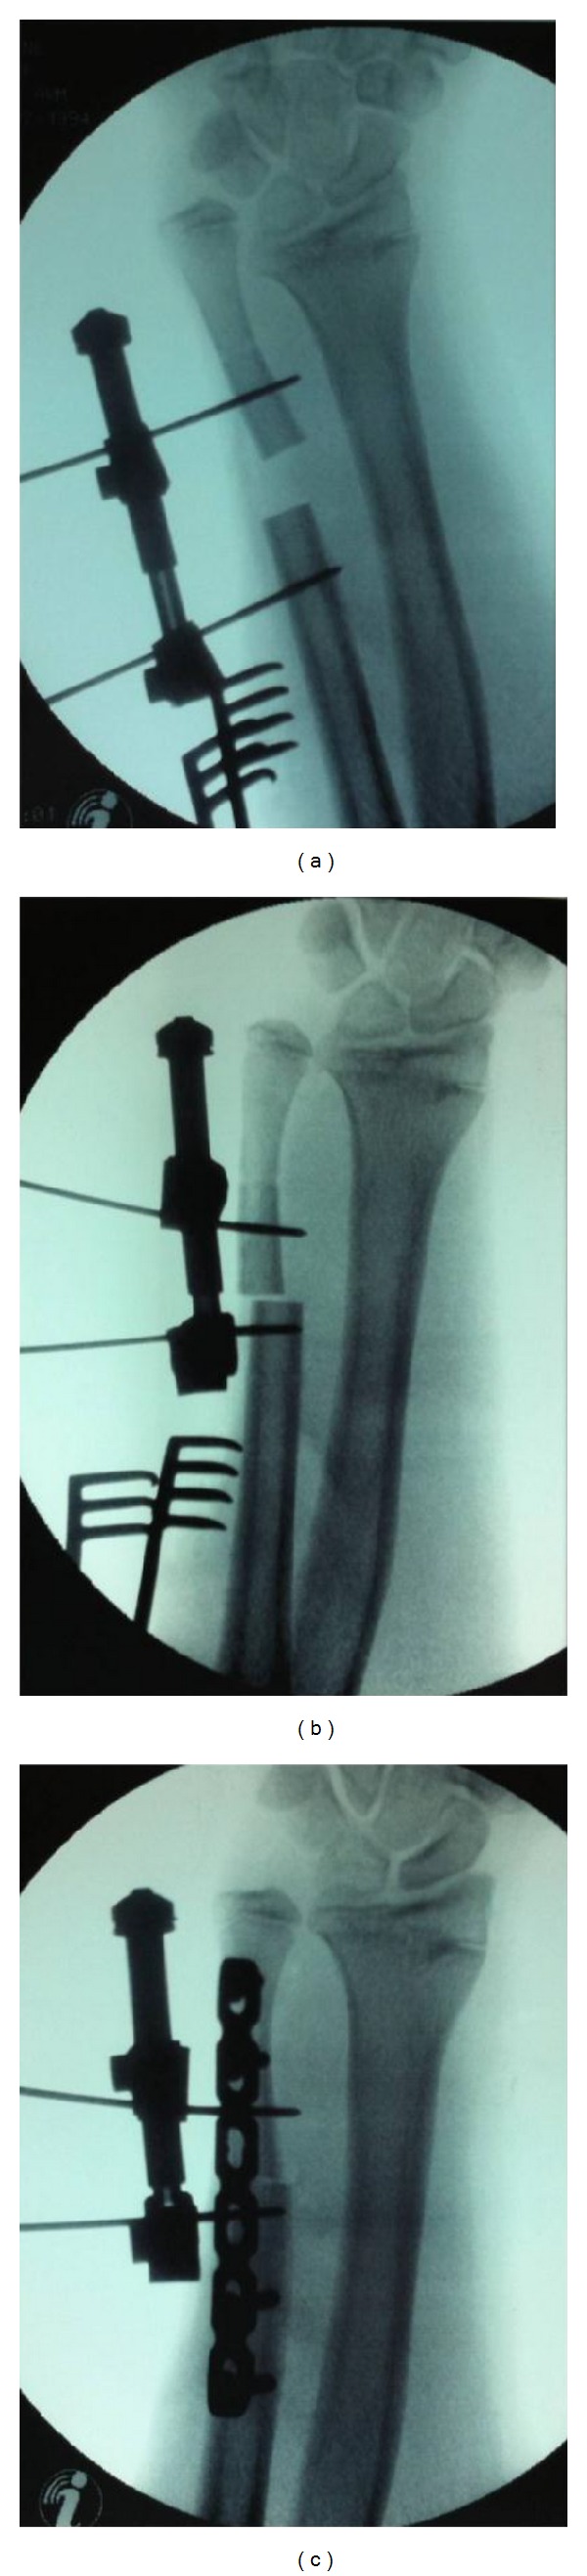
Intraoperative screening radiographs demonstrating (a) ulnar osteotomy with external fixator in situ, (b) reduction of the osteotomy bone ends, note the deformation of the Kirschner wires, (c) fixation of the osteotomy with a plate.
